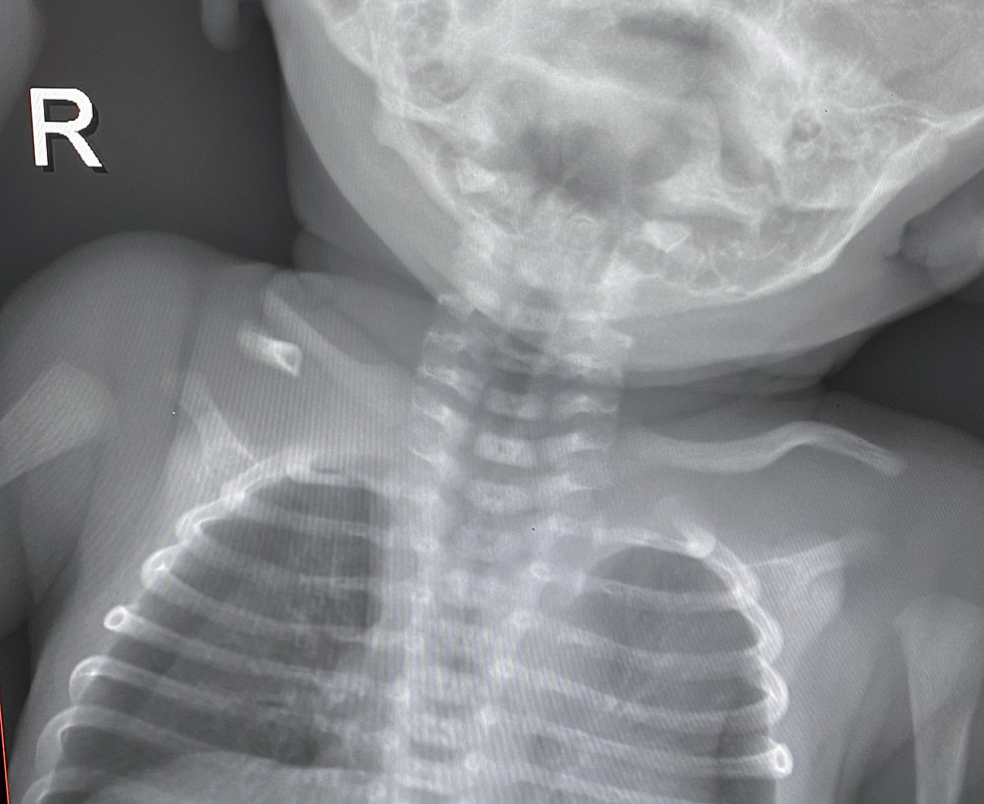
X-ray demonstrating congenital pseudarthrosis of the right clavicle.
